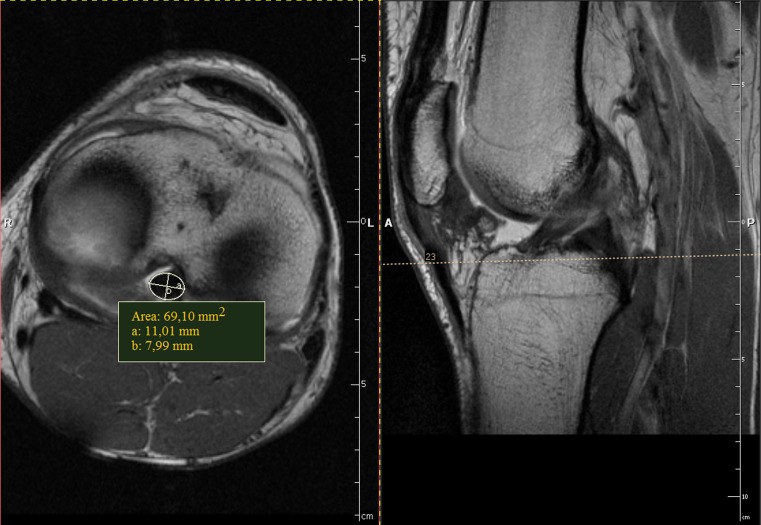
T1-weighted MR images with intraarticular administration of contrast medium. *Left side*: measurement of posterior cruciate ligament (PCL) surface area using a modifiable ellipsoid on axial section. *Right side*: measurements level (*dashed line*) on sagittal section
